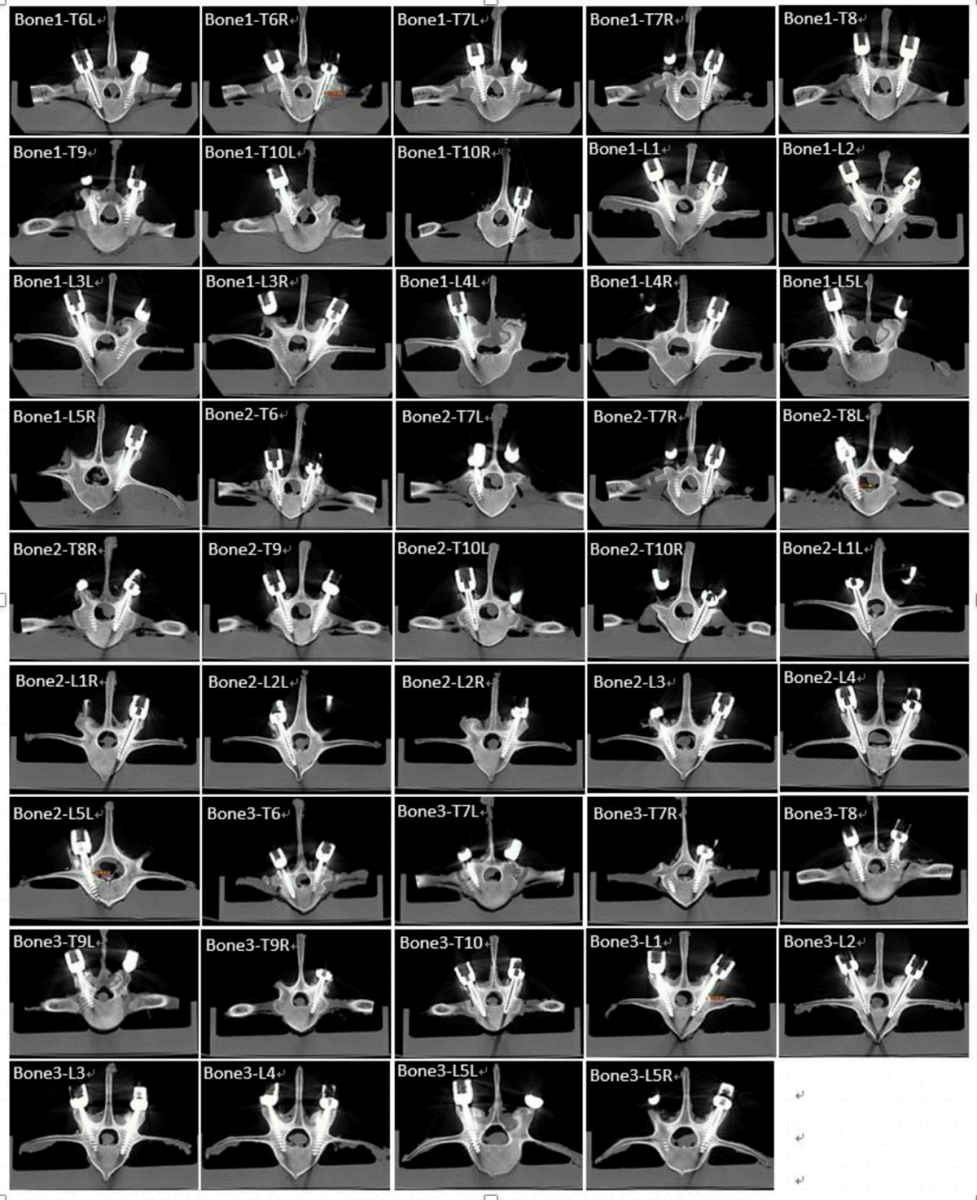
Screw position accuracy measurements determined from postoperative CT scans.
